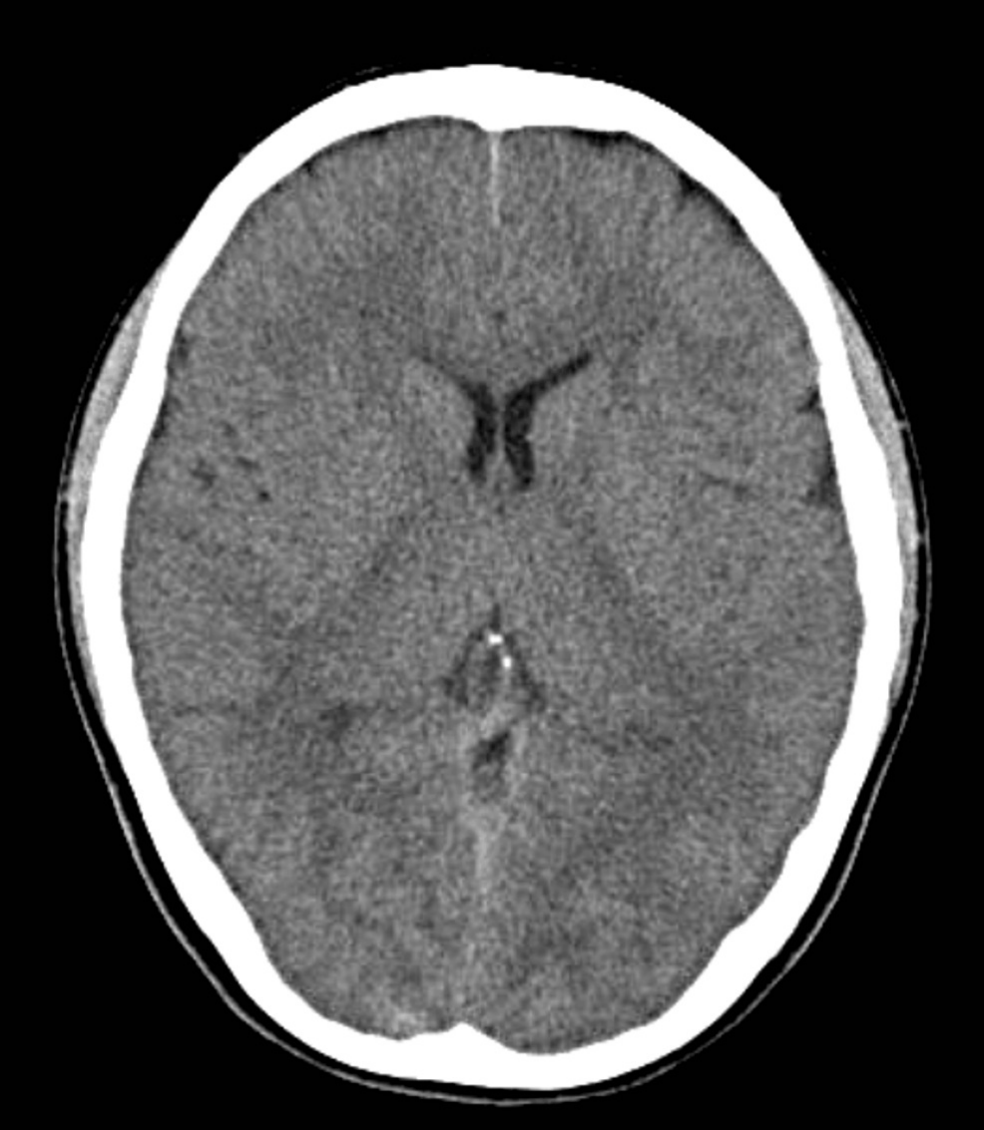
Head computed tomography performed on admission, showing an absence of calcification of the basal ganglia
